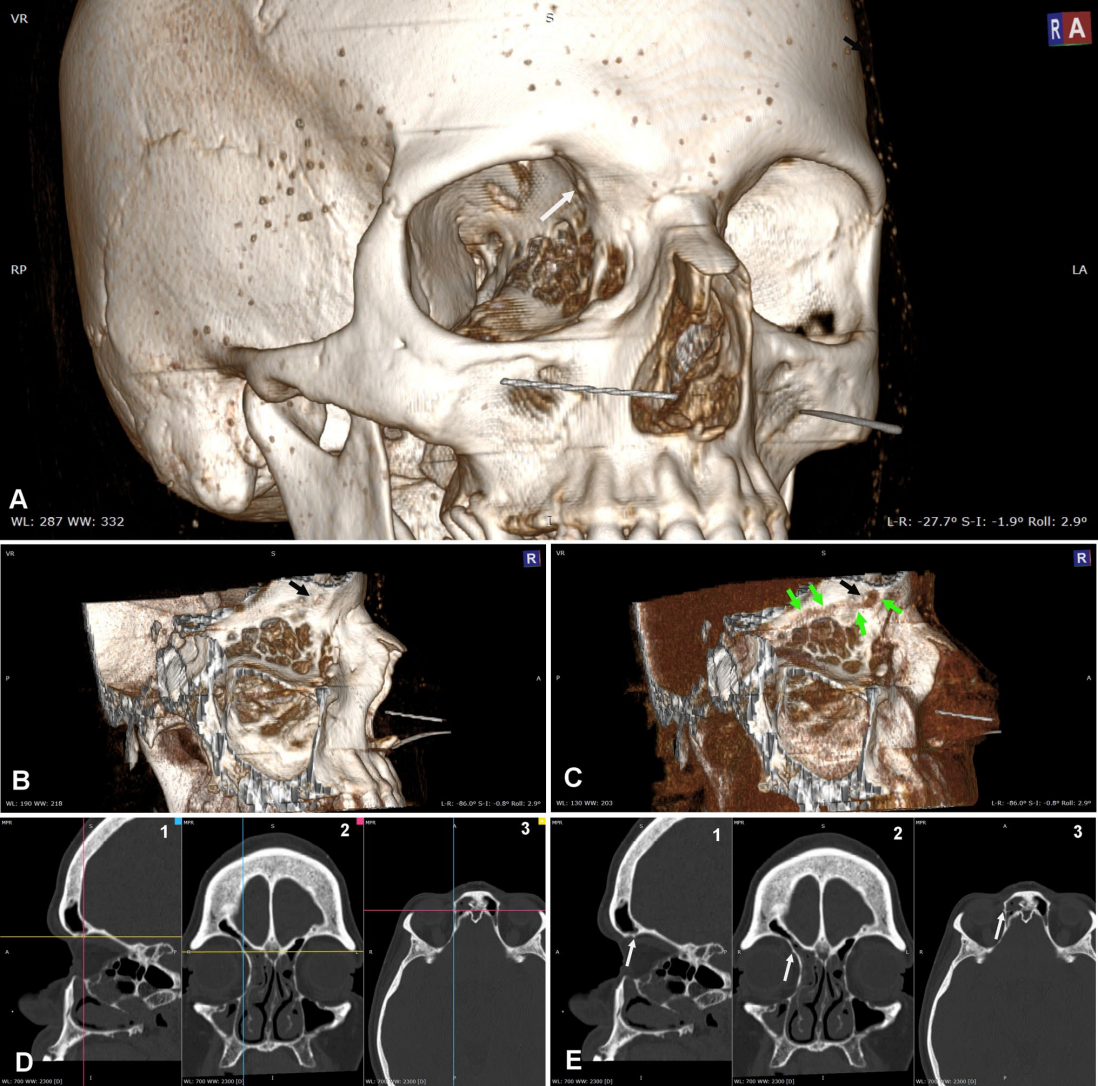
CT of a right orbit with a trochlear tubercle (TT_1_). A A TT_1_ can be seen within the orbit (arrow). B, C Lateral view of the orbit with the lateral wall and the orbit content clipped away for a better visualization of the medial wall and of the TT_1_ (black arrow). The adjustment of the image window in C allows to see the shade of the superior oblique muscle and tendon that courses under the TT_1_. D, E The same TT_1_ can be seen in MRP. D demonstrate the orientation of the planes intersecting TT_1_. D1 shows the orientation of the frontal (magenta) and axial (yellow) planes as seen on the sagittal plane; D2 shows the orientation of the sagittal (blue) and axial (yellow) planes as seen on the frontal plane; D3 shows the orientation of the frontal (magenta) and sagittal (blue) planes on the axial plane. E is the same image seen in D without showing the planes to better visualize TT_1_ (white arrows) on the sagittal (E1), the frontal (E2) and the axial (E3) planes
